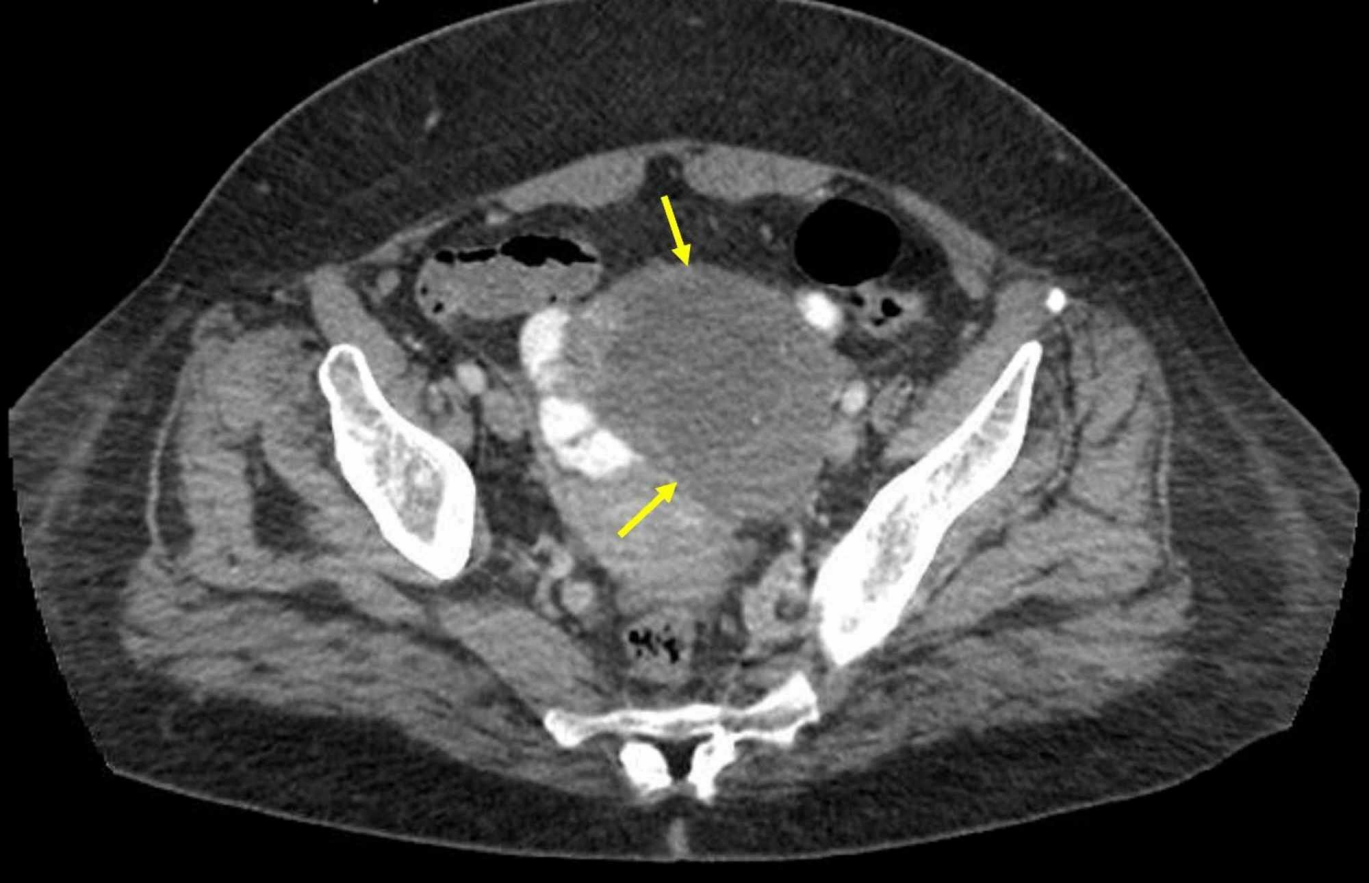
Pelvic CT showing semi solid/semi cystic left ovarian mass measuring 8.3 cm (yellow arrows).
